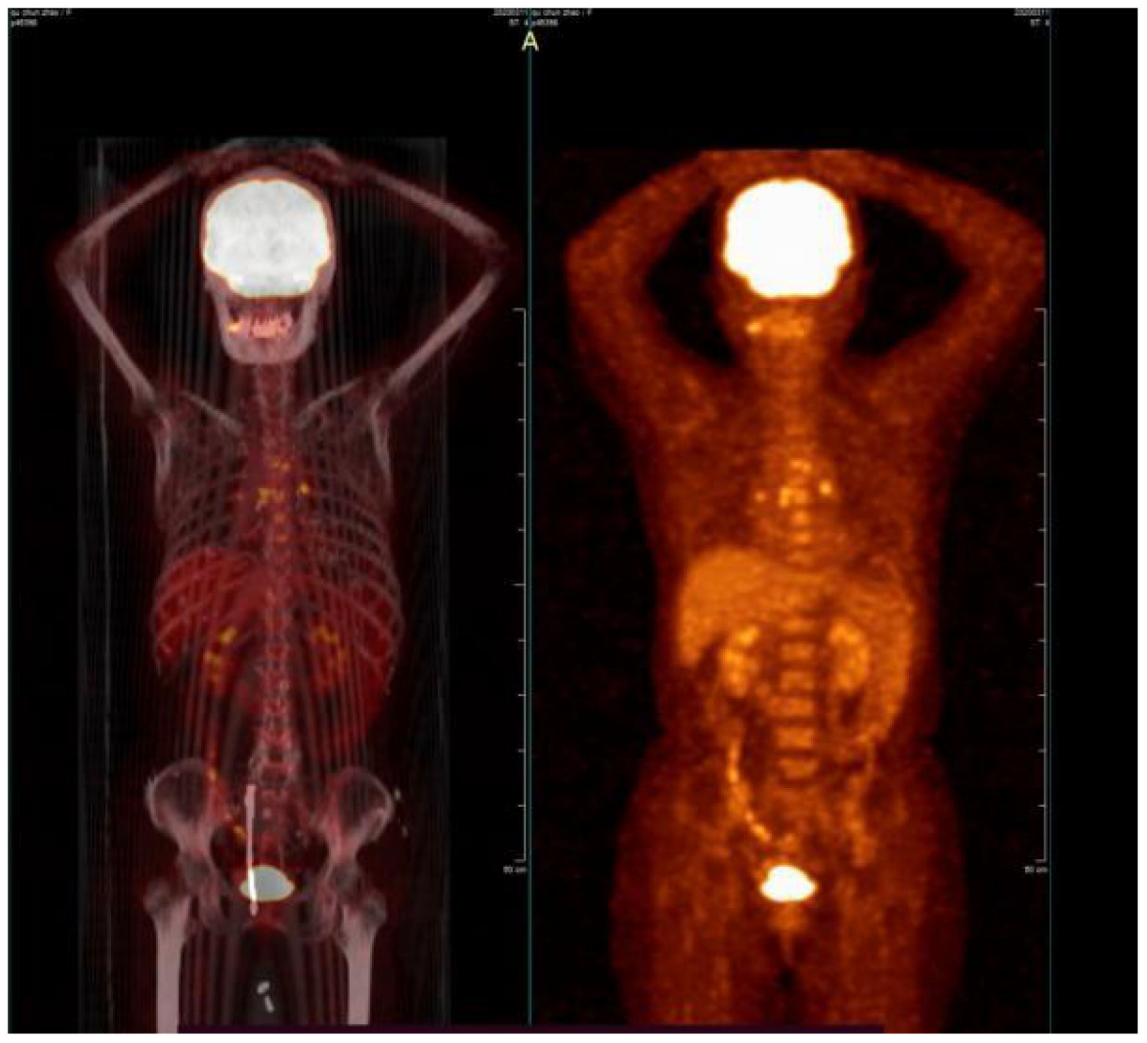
PET-CT before treatment showed that the mass was located in the cervix.
